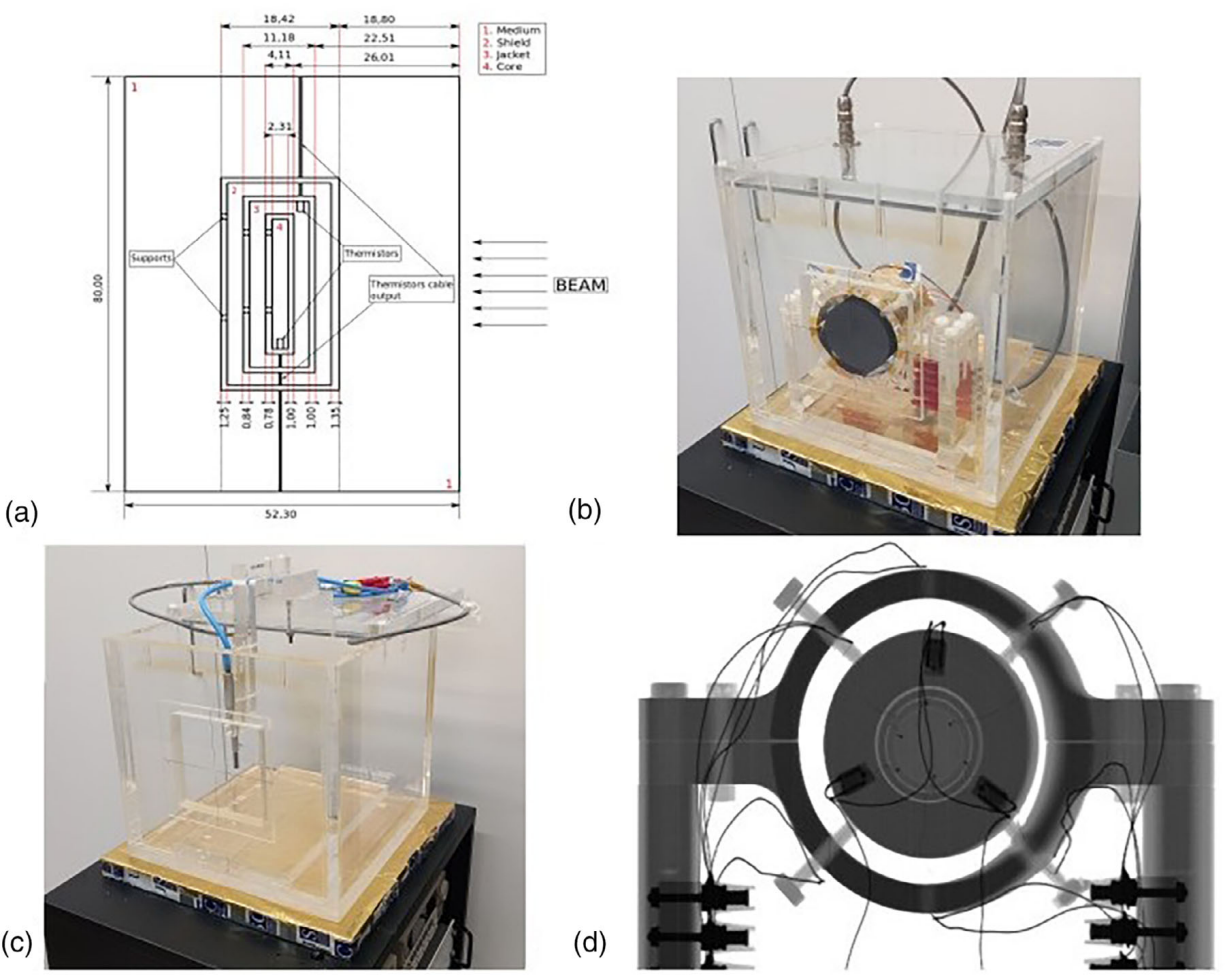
(a) Dimensions of graphite bodies. Beam field size is 10 × 10 cm. (b) Image of the calorimeter inside the empty tank without a polystyrene thermal shield. (c) Tank without calorimeter with ion chamber positioned for kQ determination or calibration. d) Radiograph of the calorimeter and its support.
